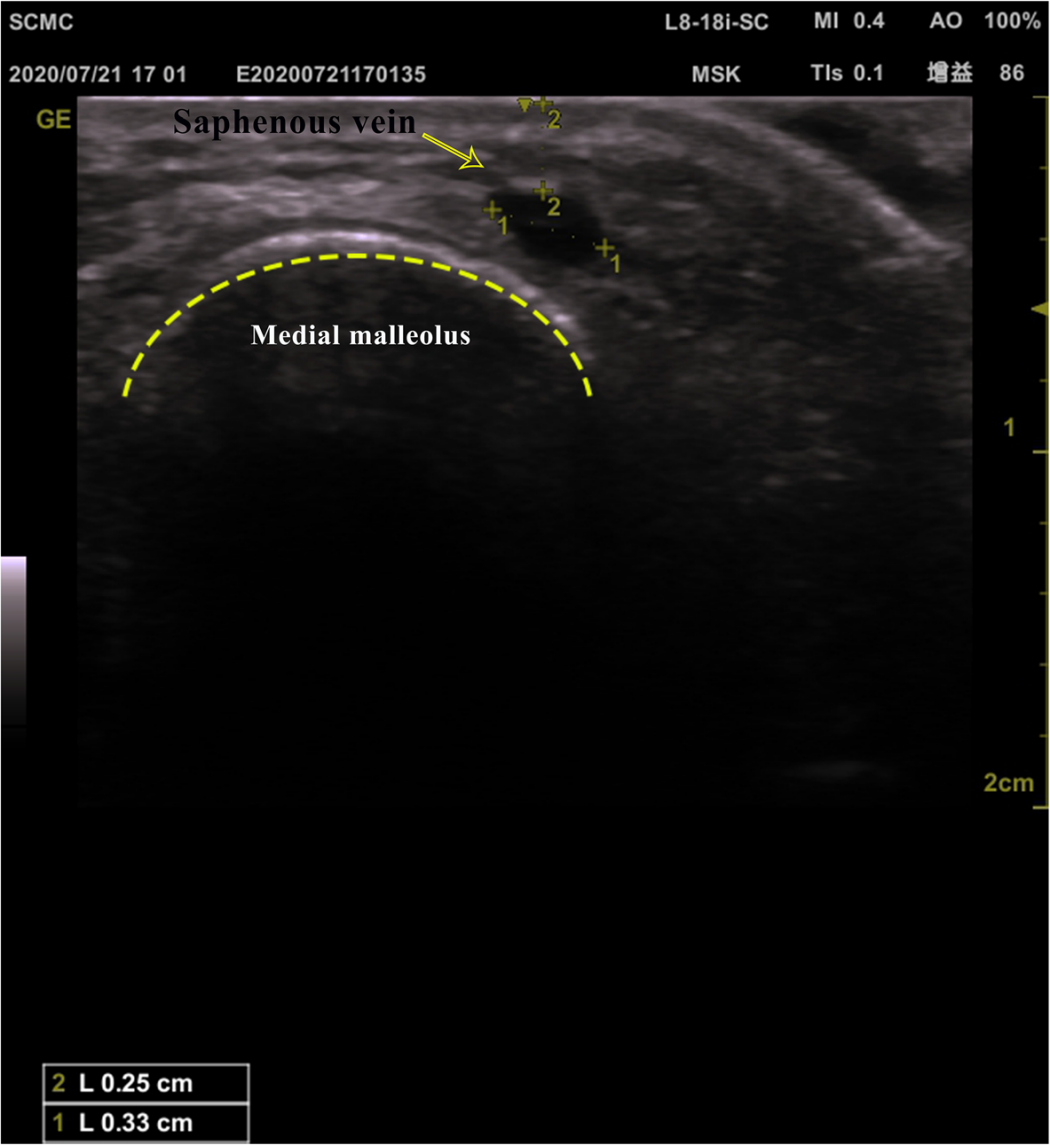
Legend of ultrasonic measurement of depth and width of a saphenous vein, symbol 1 is the width; symbol 2 is the depth
